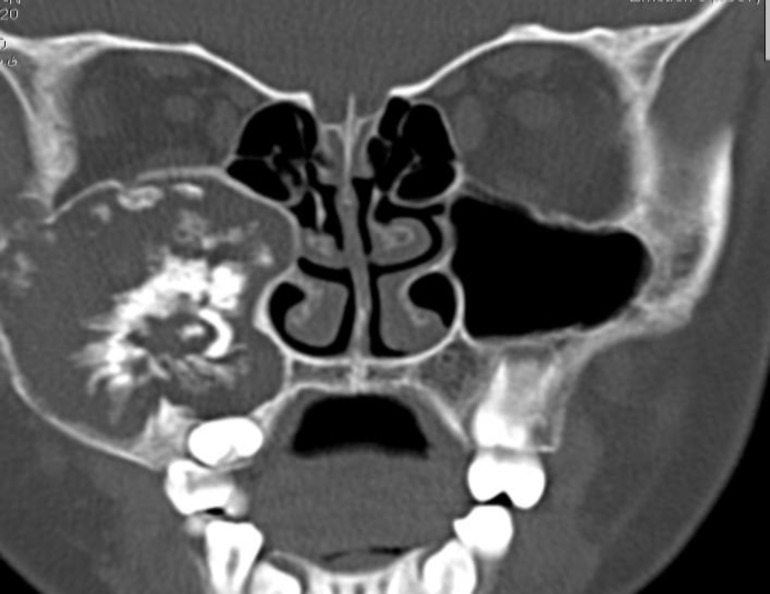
The coronal CT scan demonstrates a very large mixed tumor in the right maxillary sinus. Notice to the size of the lesion and extending to eye floor and wall of the nose
